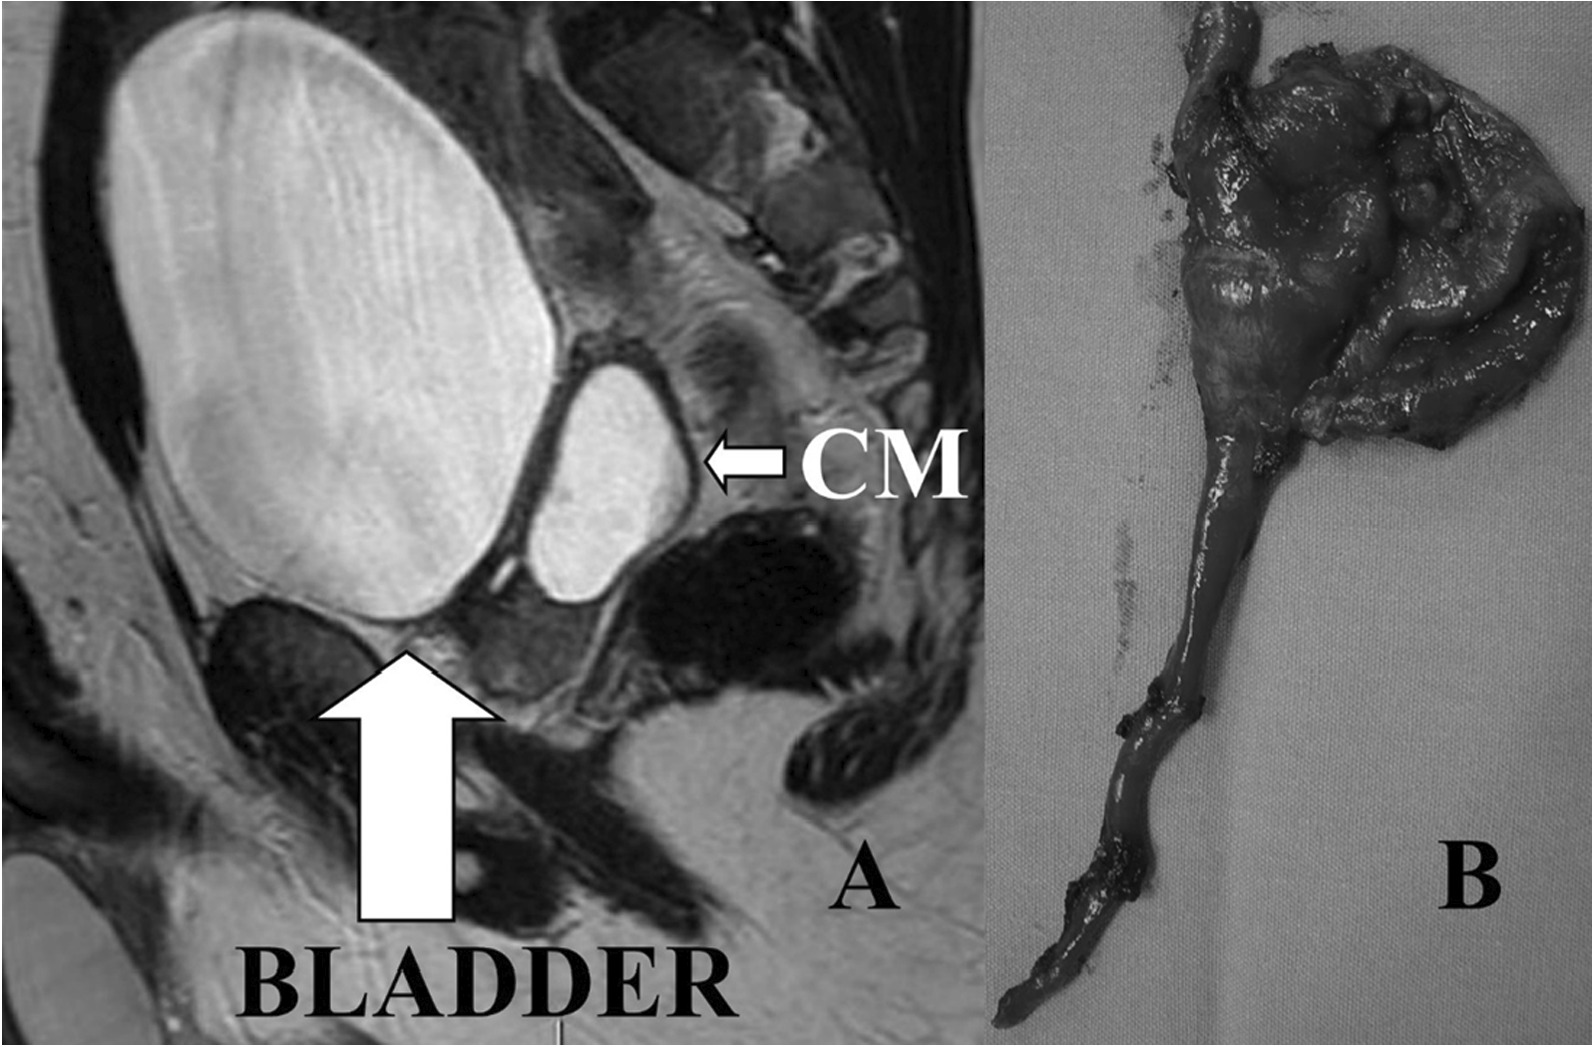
A MRI image of the cystic mass (CM). B Cystic mass originating from the deferent duct
